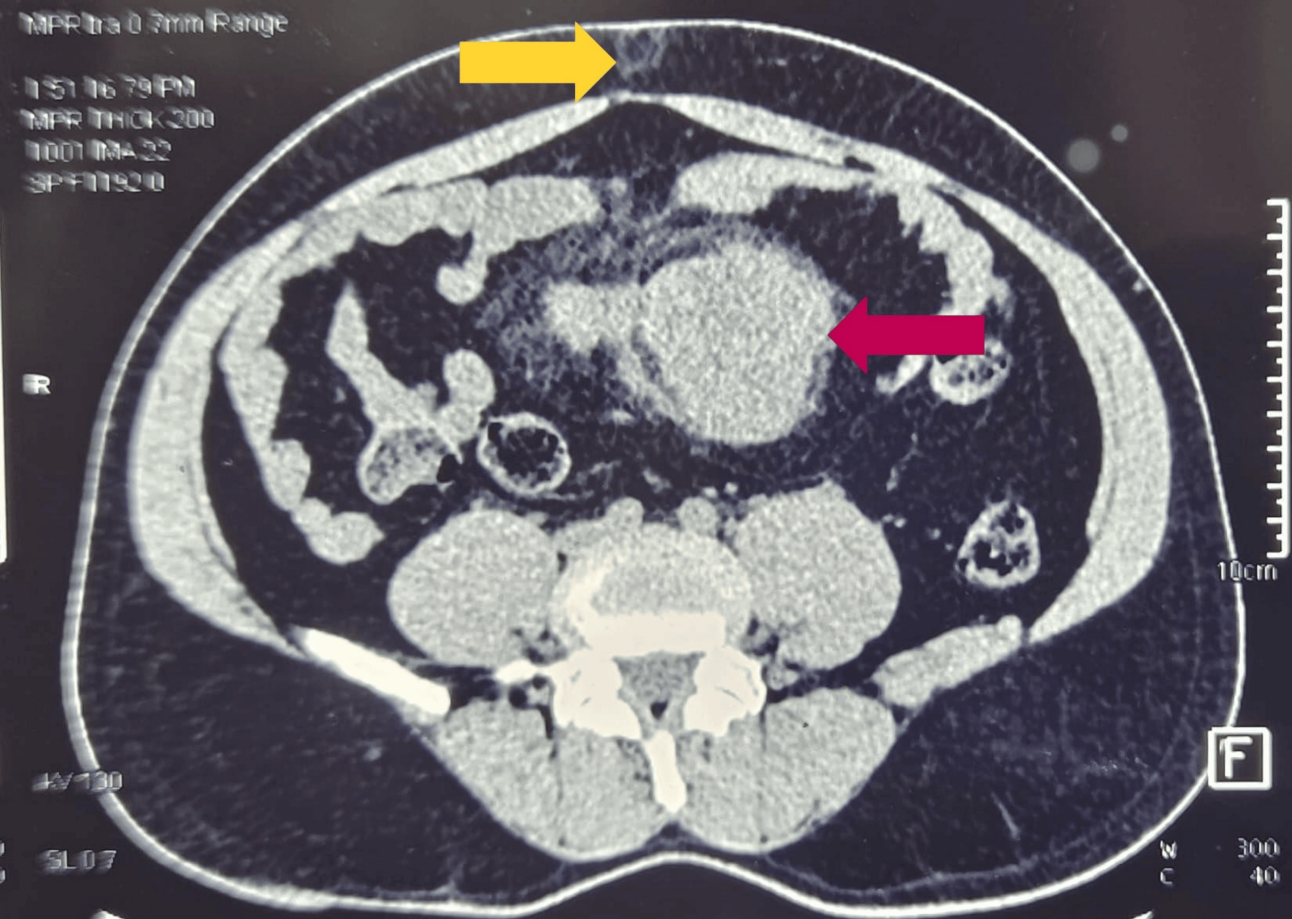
Transverse section of the abdominal CT scan, showing the umbilical hernia (yellow arrow) and the tumor (red arrow) CT: computed tomography
